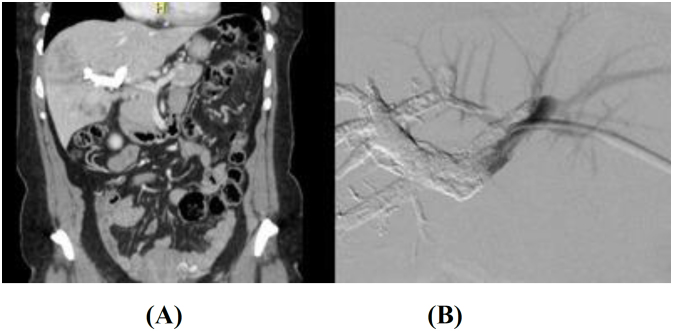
(A) Computed tomography scan revealed thrombosis of left portal vein small branches (B) secondary to migration of embolizing material inside left portal vein.
